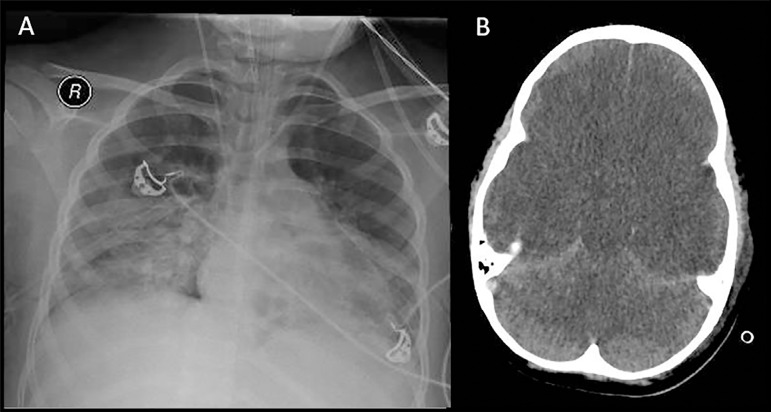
Imaging exams. (A) Chest radiograph showing diffuse pulmonary infiltrate. (B) Brain computed tomography showing cerebral edema.
